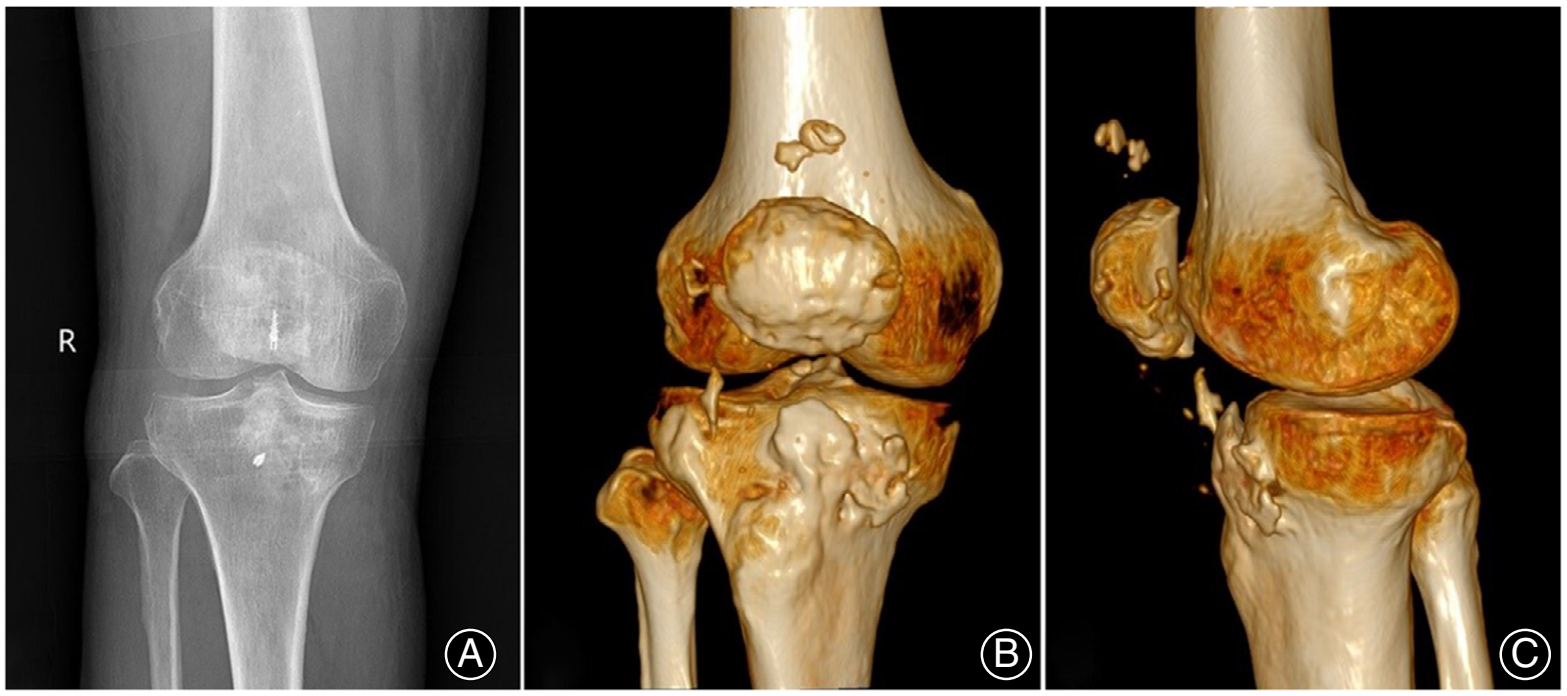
Postoperative anteroposterior radiograph (A) and three‐dimensional reconstruction CT images (B, C) revealing nearly complete debridement of the ossified patellar tendon. Note the suture anchors placed in the patella and tibia for the reconstruction of patellar tendon.
